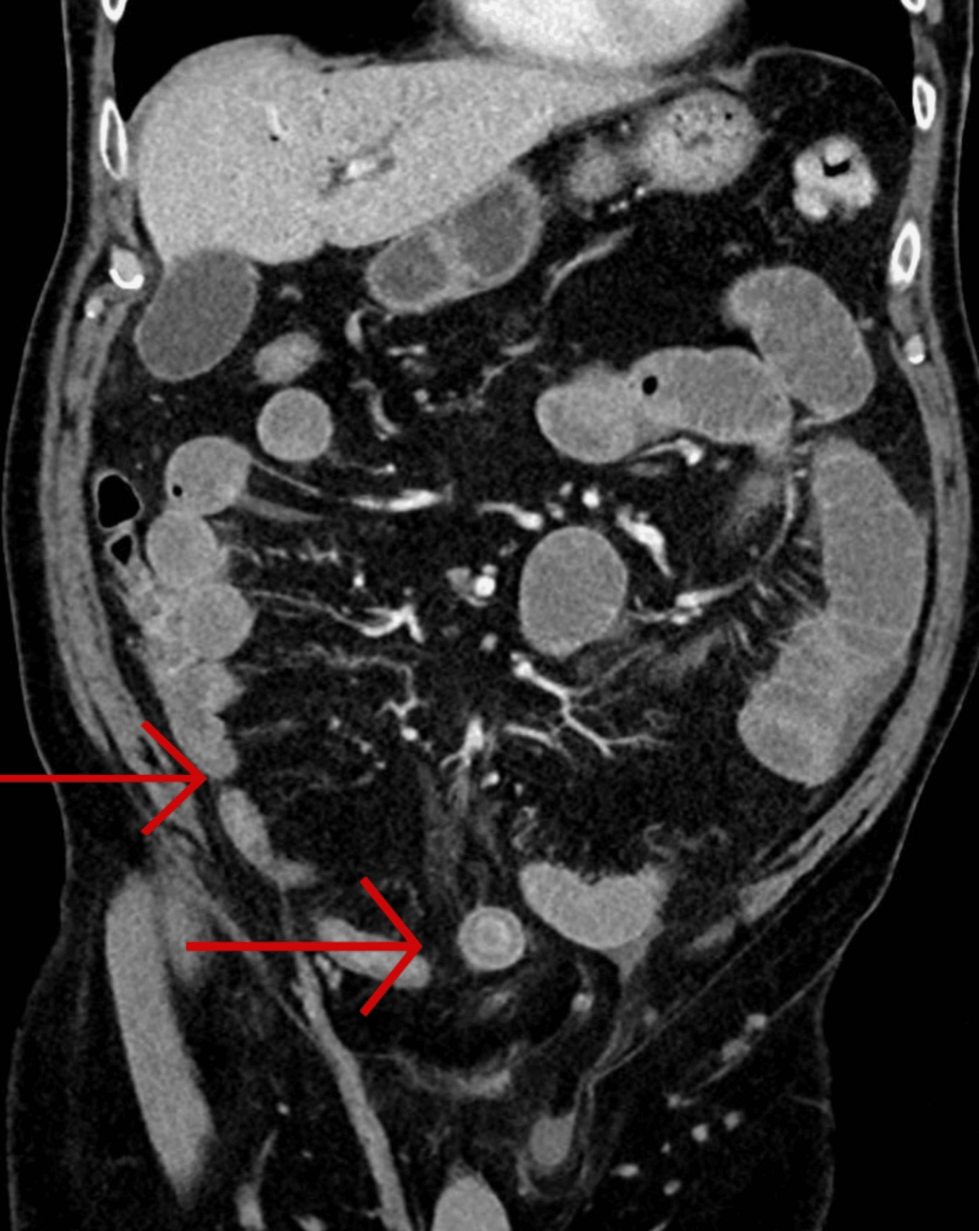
CT abdomen and pelvis with contrast Imaging demonstrating small bowel dilatation with collapsed ascending colon and large bowel with suspicion of gallstone ileus (red arrows)
